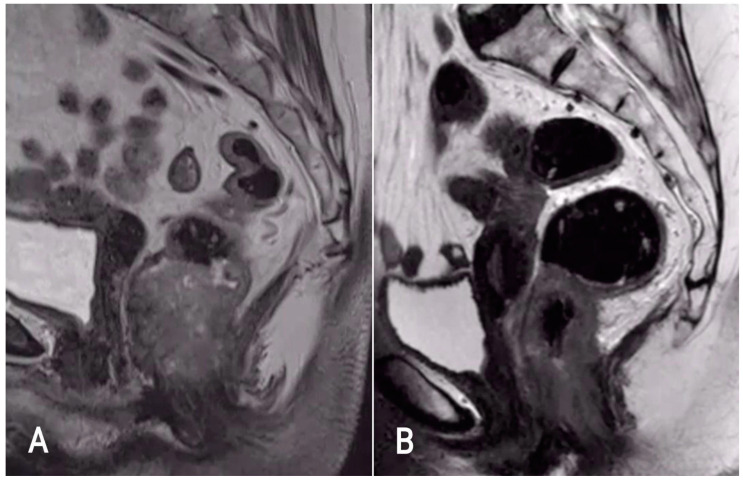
Sagittal T2-weighted MR images of rectal adenocarcinoma (A) and anal squamous-cell carcinoma (B). Both typically display intermediate signal intensity on T2-weighted imaging.
